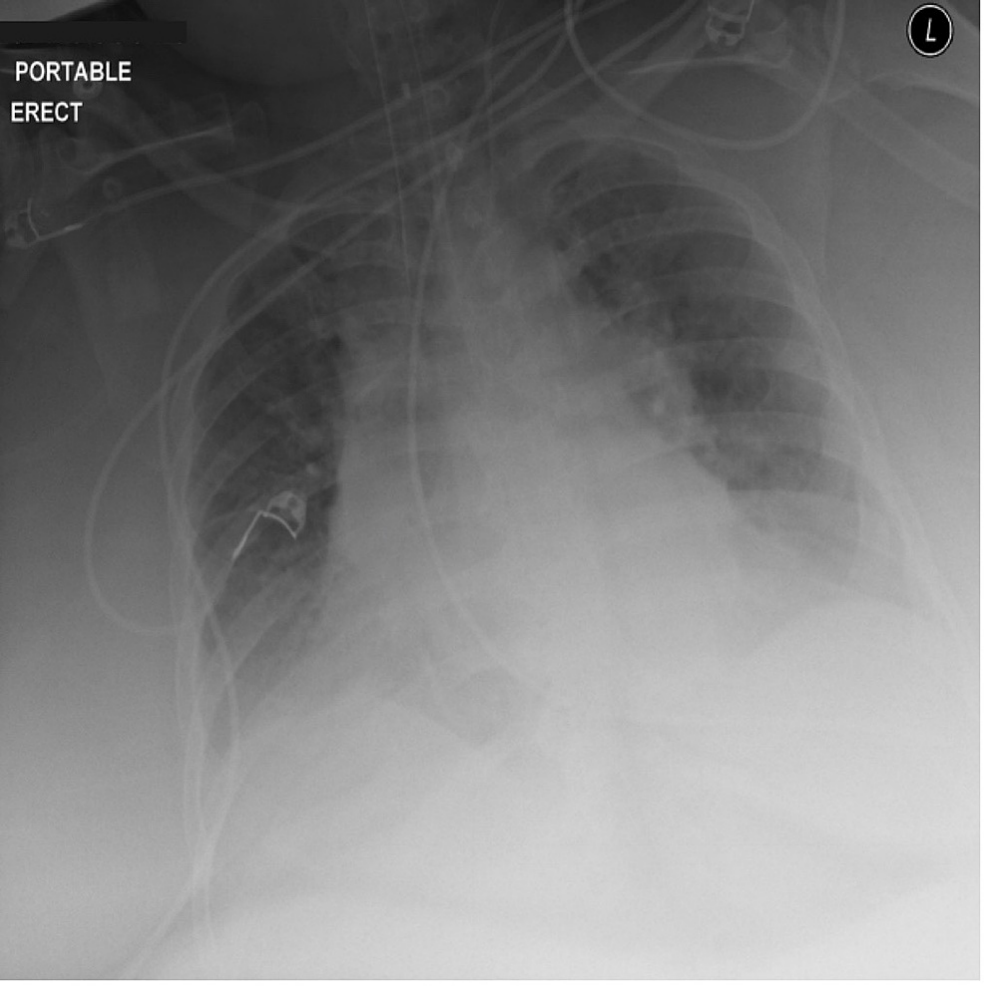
Portable anteroposterior chest X-ray showing hyperinflation of lungs bilaterally. Cardiac silhouette and other radiographic landmarks are difficult to appreciate secondary to body habitus.
